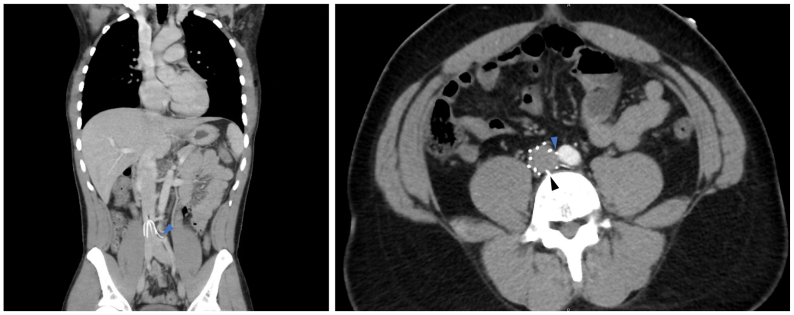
Preoperative computed tomography (CT) scan showing the inferior vena cava (IVC) filter struts eroding into the L4 vertebral body (black arrowhead) and abutting the proximal right common iliac artery (blue arrowhead).
